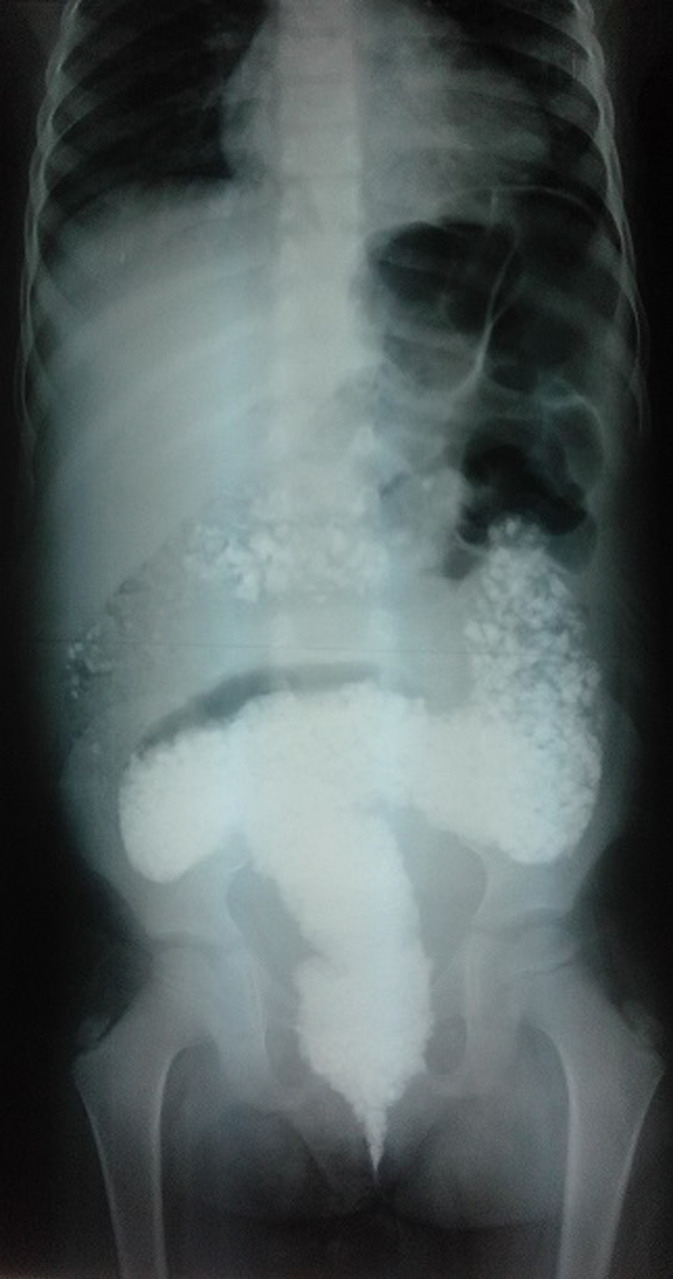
X-ray of the abdomen showing gravel inside the large bowel and rectum
